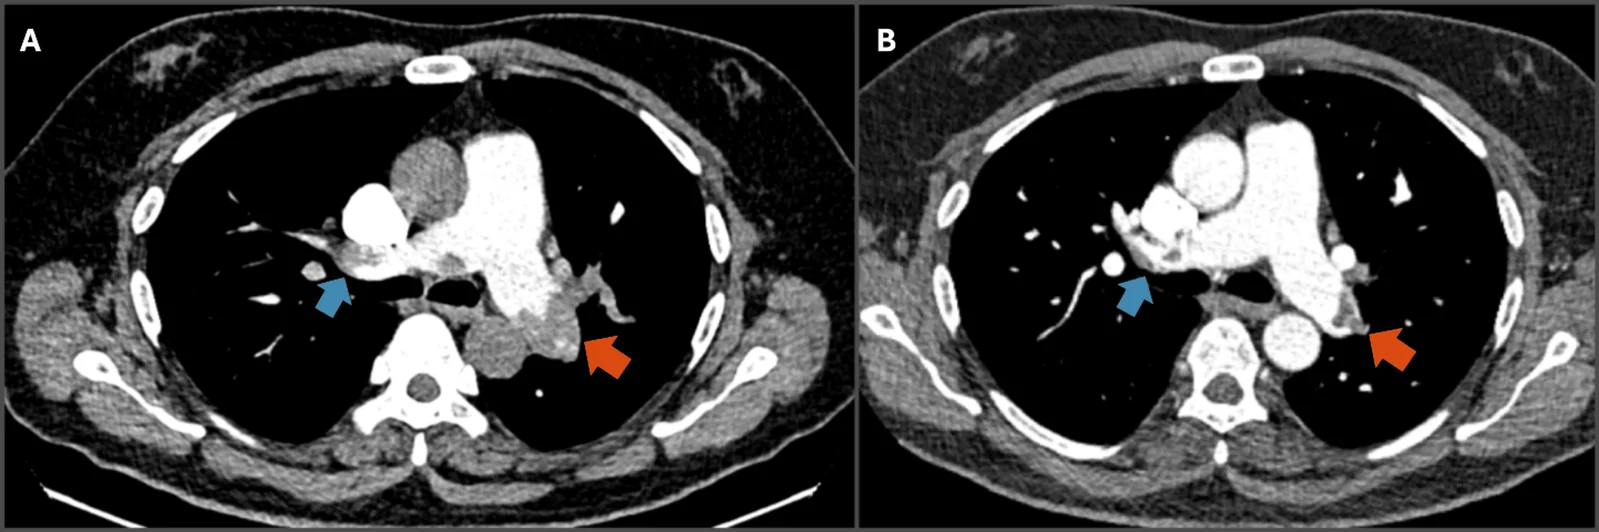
Case 4: A. Pre-thrombolysis axial CT scan with contrast shows bilateral pulmonary embolism occluding the right main pulmonary artery (blue arrow) and the left main pulmonary artery (orange arrows). B. Post-thrombolysis axial CT scan shows significant recanalization of the right main pulmonary artery (blue arrow) and the left pulmonary artery (orange arrow).
